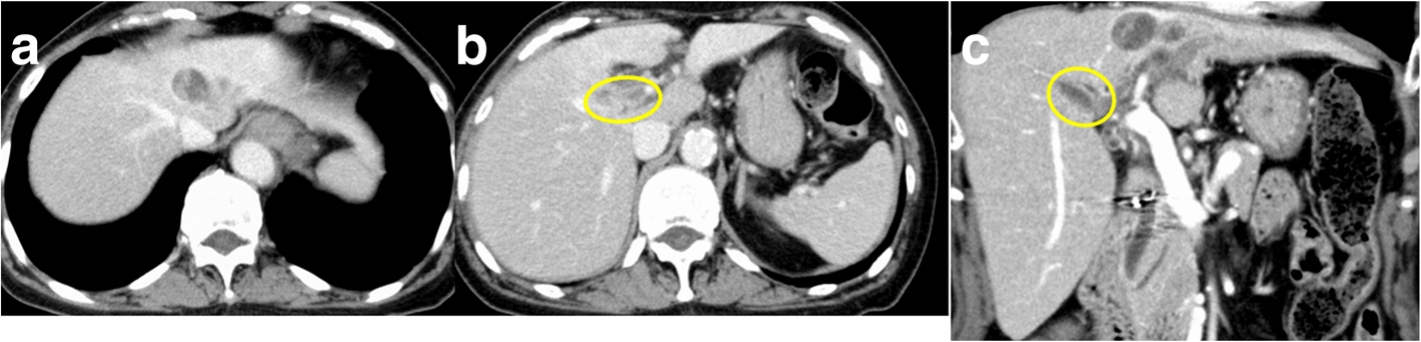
Contrast-enhanced CT after preoperative PIHP: CT showing the reduction of the main tumor volume (a) and no enhancement of the PVTT (*yellow circle*) after PIHP (b, c)
